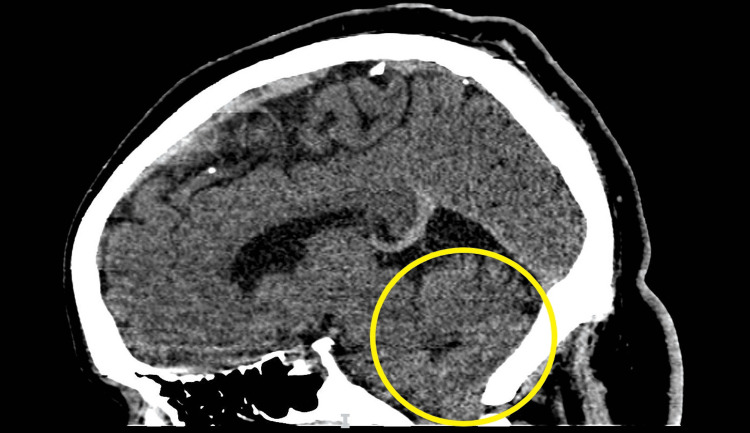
CT head demonstrating cerebellar tonsillar herniation (sagittal view) Yellow circle: Downward cerebellar tonsillar herniation extending below the foramen magnum
